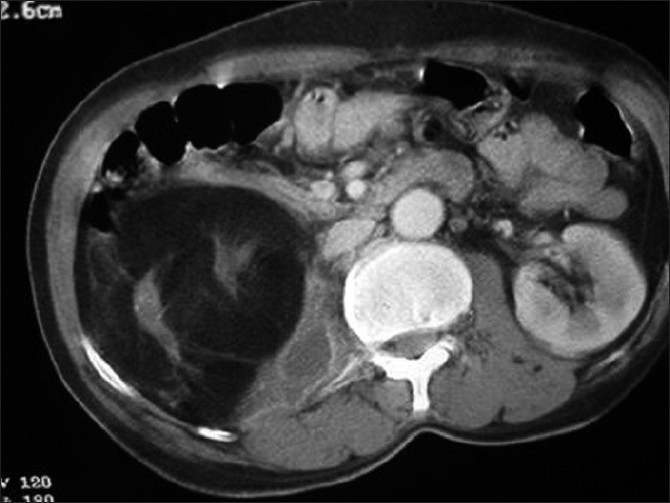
Contrast-enhanced CT of abdomen shows replacement of right kidney by fat with a large central hyperdense calculus. A small posterior perinephric collection is noted extending into the adjacent psoas. Thin residual strands of renal tissue are noted
